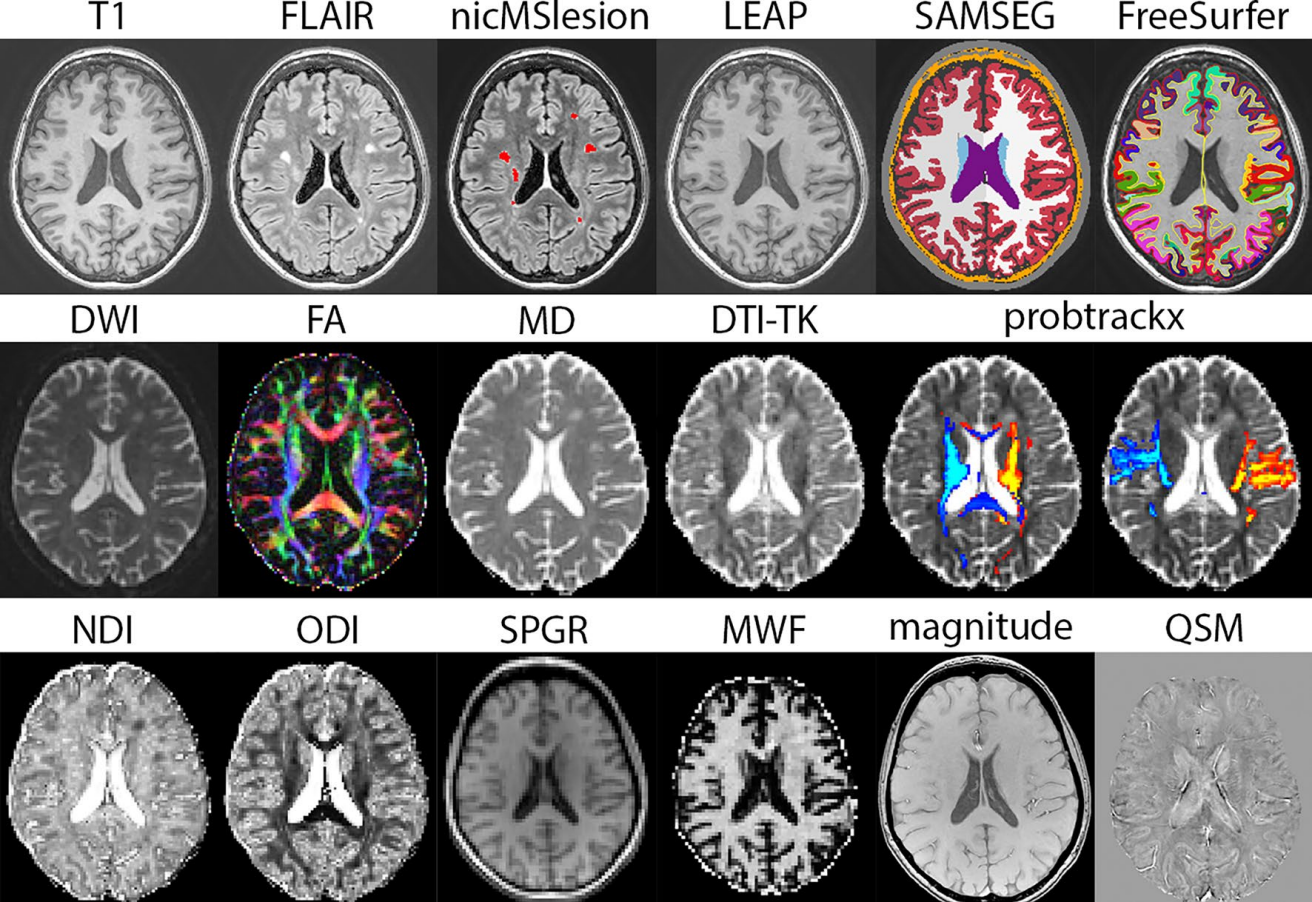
Overview of MR imaging analysis. Top row: T1 and FLAIR images were used to create a lesion mask (nicMSlesions) and lesion filled (LEAP) image, which was used as input for longitudinal SAMSEG for subcortical segmentation and longitudinal FreeSurfer for cortical parcellation. Middle row: multi-shell diffusion weighted images (DWI) were used to obtain FA and MD in the WM mask. A tensor image from DTI-TK was used to register FreeSurfer ROIs, and probtrackx was used for tractography (example from thalamus tracts and postcentral tracts). Bottom row: NODDI was used to obtain NDI and ODI maps; SPGR images were used to create MWF maps; magnitude and phase (not shown) images were used to create QSM maps
